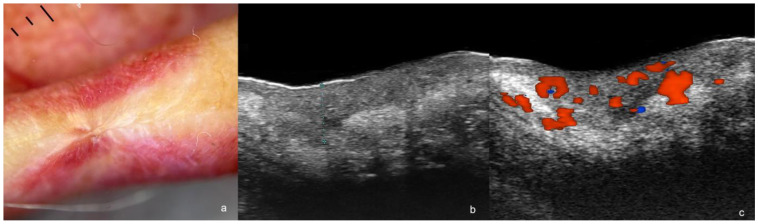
(a–c). Post-operative recurrence of squamous cell carcinoma. (a). Dermoscopy shows a lower lip scar. (b,c). Ultrasound images at 71 MHz ((b), greyscale and (c), color Doppler, transverse views) present a hypoechoic dermal and hypodermal structure (thickness marked in (b)) with irregular borders and increased vascularity (c).
